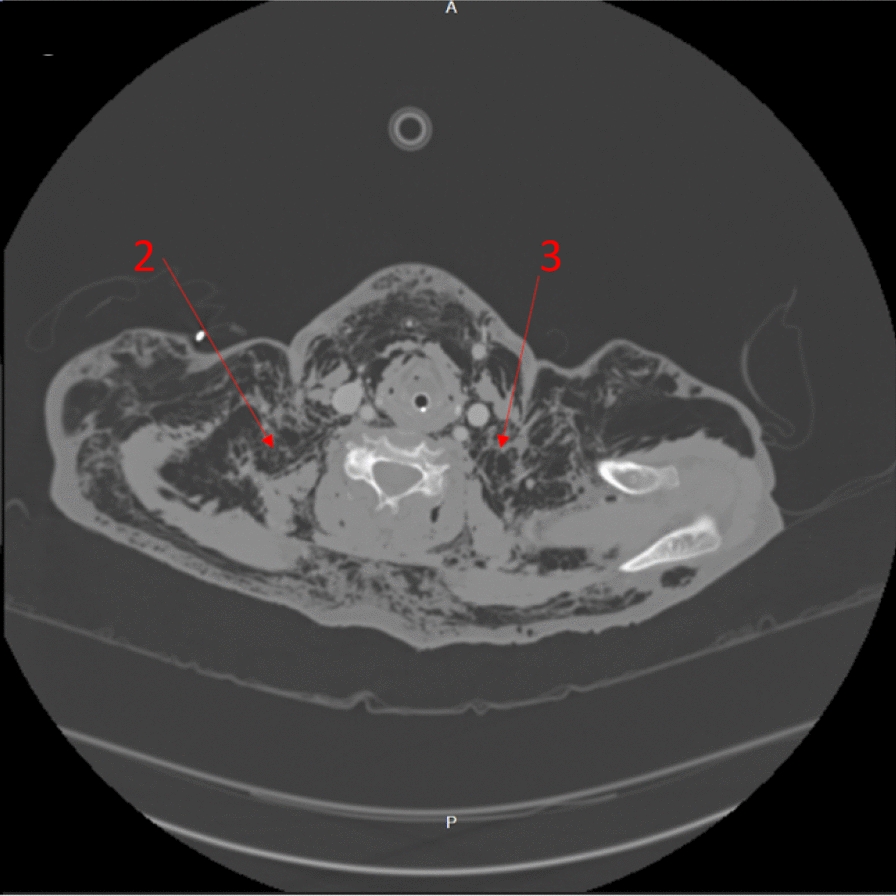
Axial computed tomography showing emphysema penetrating the deep neck tissues (arrows 2 and 3)
